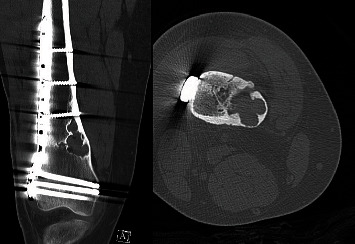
CT obtained 16 months postoperatively showing an anterior cortical defect approximately 7 cm proximal to the distal femur.
